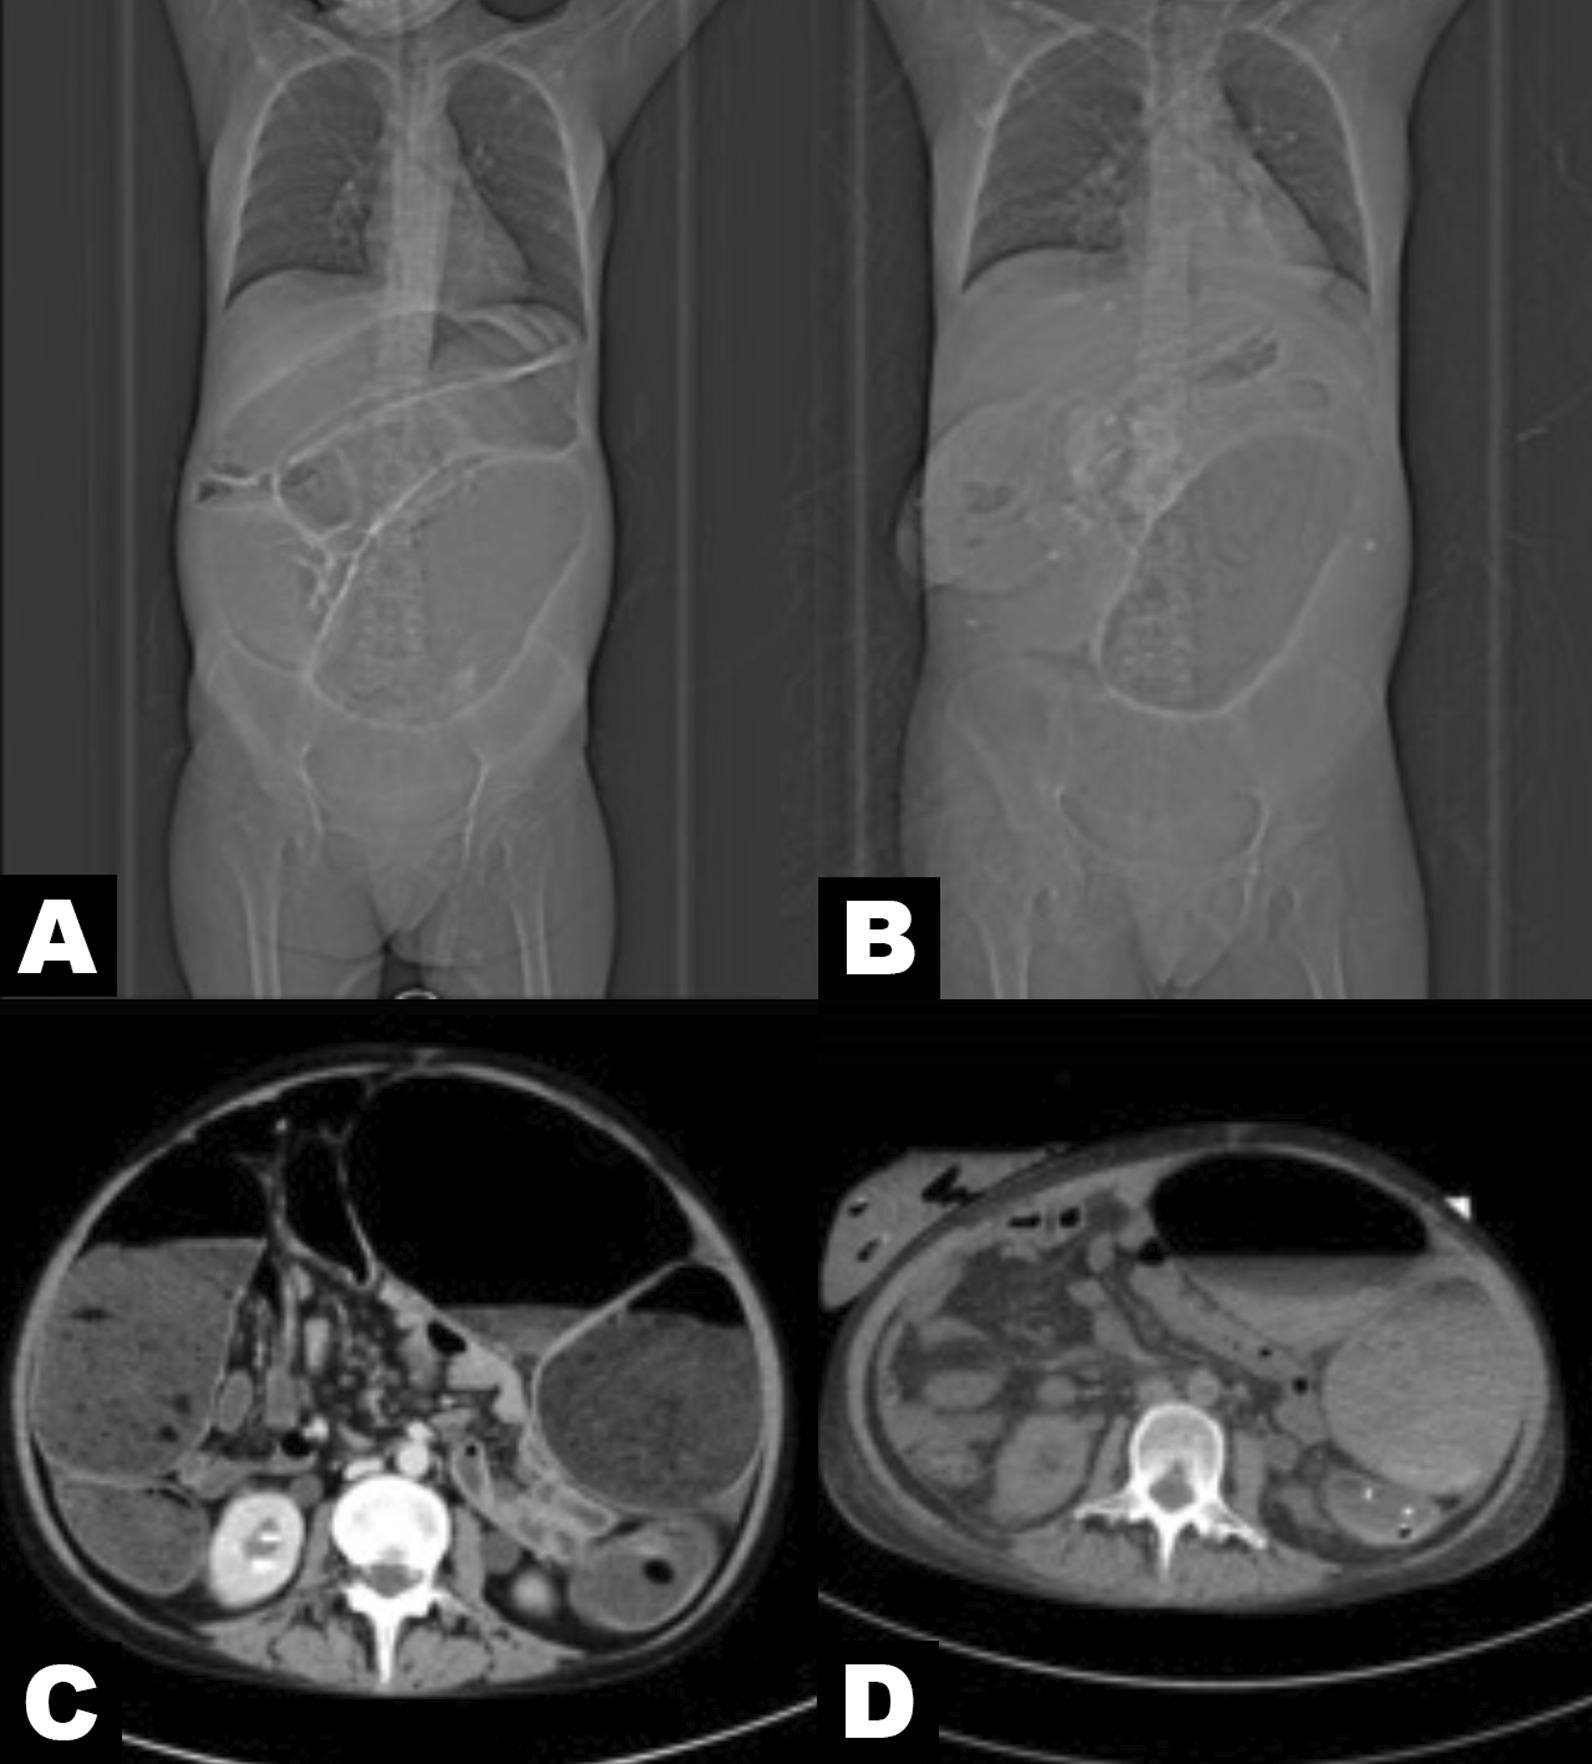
Preoperative and postoperative abdominal computed tomography scans. Compared to preoperative abdomen reconstruction (A) and axial reconstruction (C), postoperative abdomen reconstruction (B) and axial reconstruction (D) showed that the patient’s bowel dilatation had improved significantly after surgery
